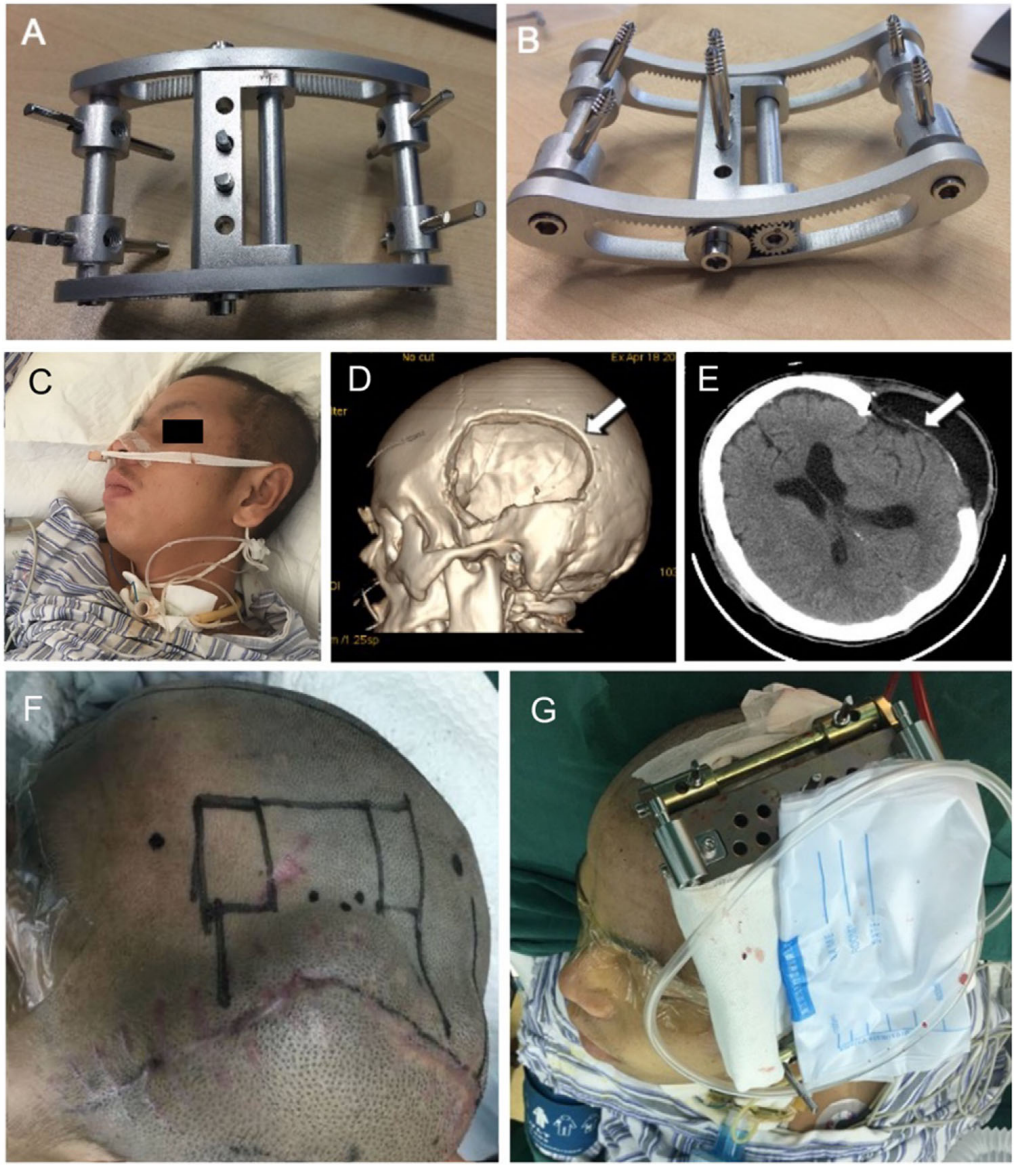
An 18‐year man suffered TBI was performed CBT surgery. (A‐B) Structure of the external fixator: anterior view A) and dorsal view B). C) Patient was in a state of mild disturbance of consciousness with a trachea intubation to maintain ventilation. D) CT reconstruction showed a bone defect in the left skull after decompressive craniectomy for about 4 months. E) Representative CT Image of horizontal position indicated large defects in parietal region. F) A bone flap was designed to be obtained in the rim of bone gap border. G) View of patient with the external fixator after surgery.
